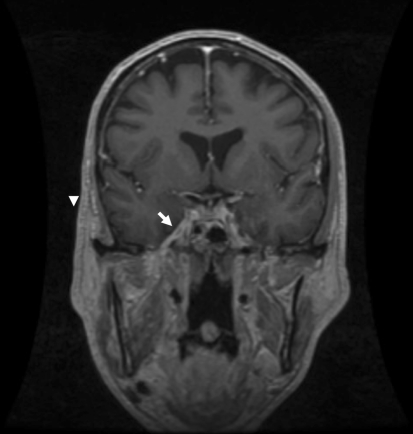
MRI coronal view shows perineural enhancement of the right maxillary nerve (*arrow*), and enhancement around cavernous sinus and the Meckel cave
